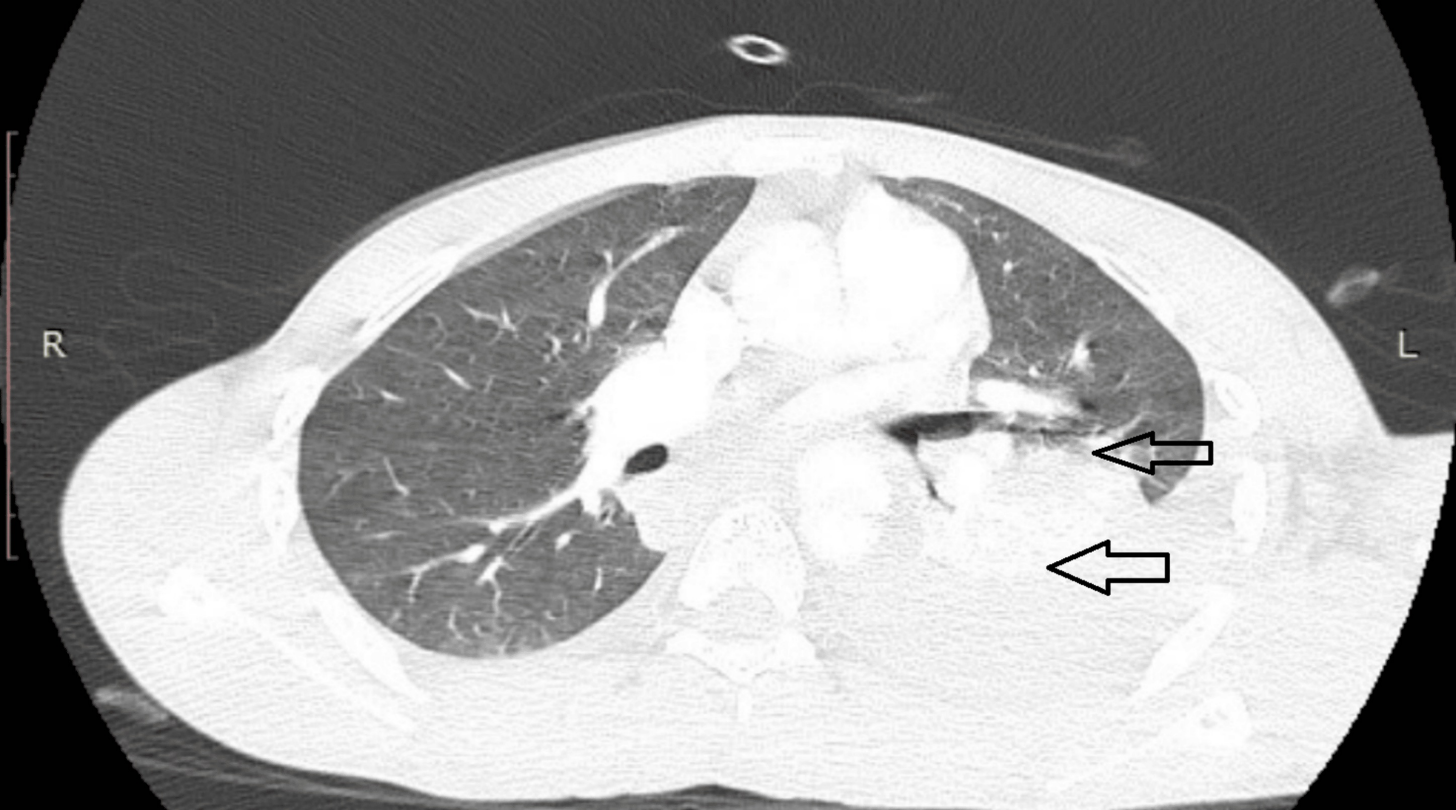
Preoperative computed tomography (CT) imaging Axial lung-window CT images demonstrating left-sided hemothorax with partial collapse of the left lung, consistent with traumatic injury following blunt chest trauma (arrows). The right lung appears relatively well aerated.
